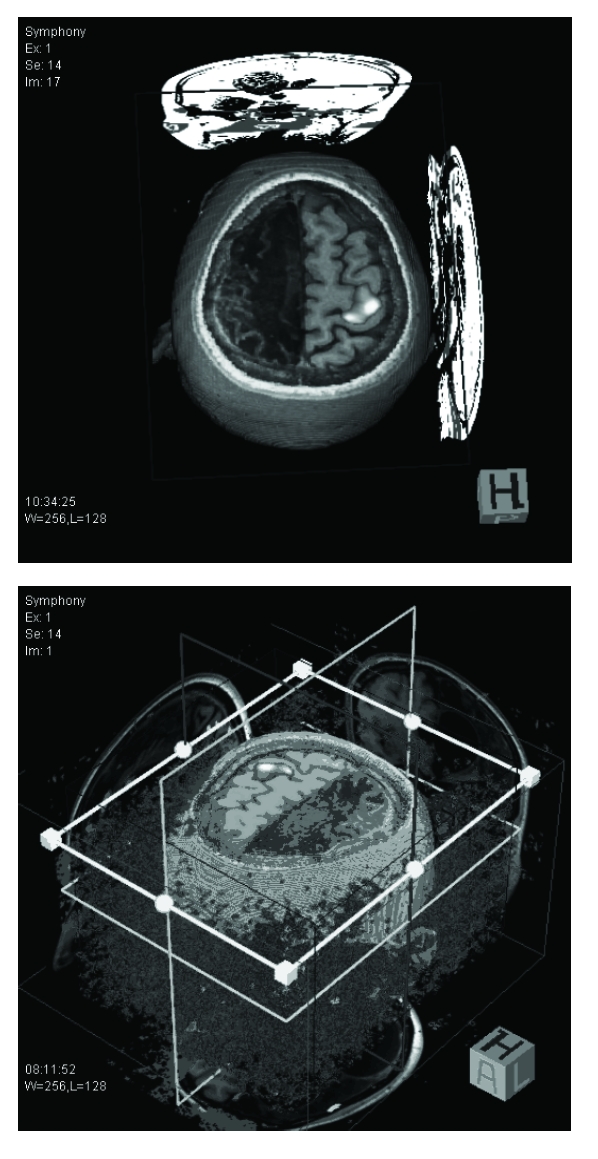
Functional MRI shows right hemisphere function on task–related brain activation during voluntary movement of patient's right leg
